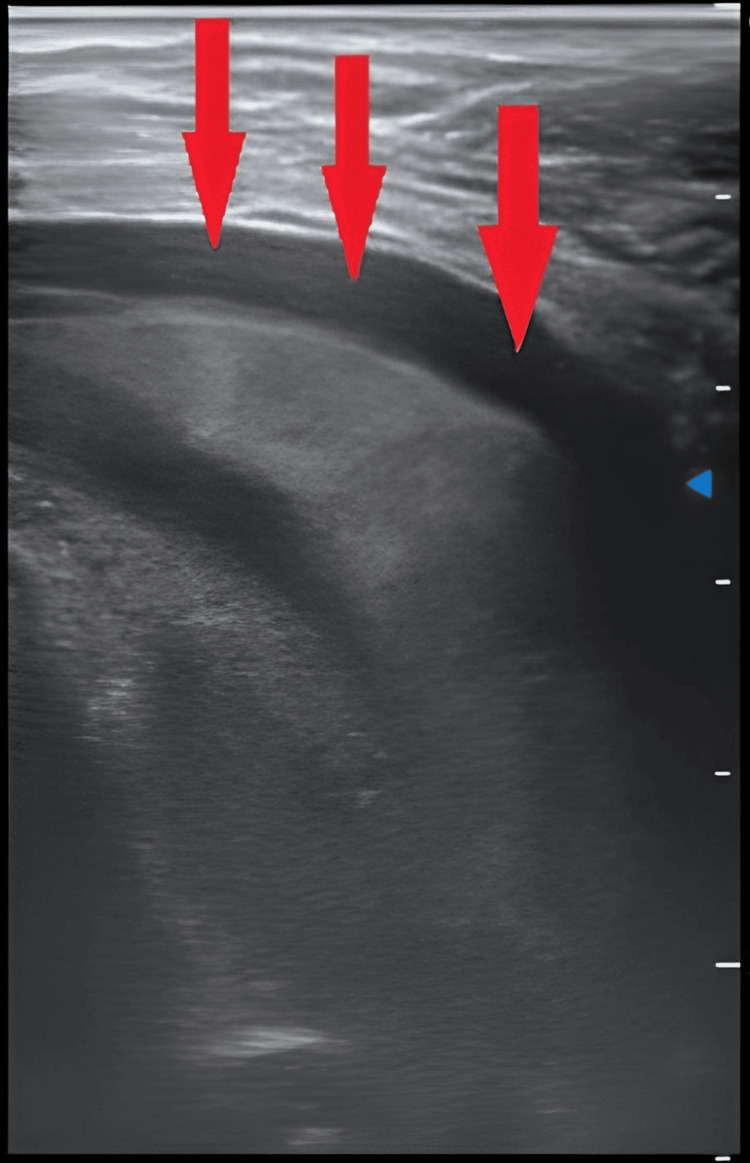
Chest X-ray showing a left pleural effusion
